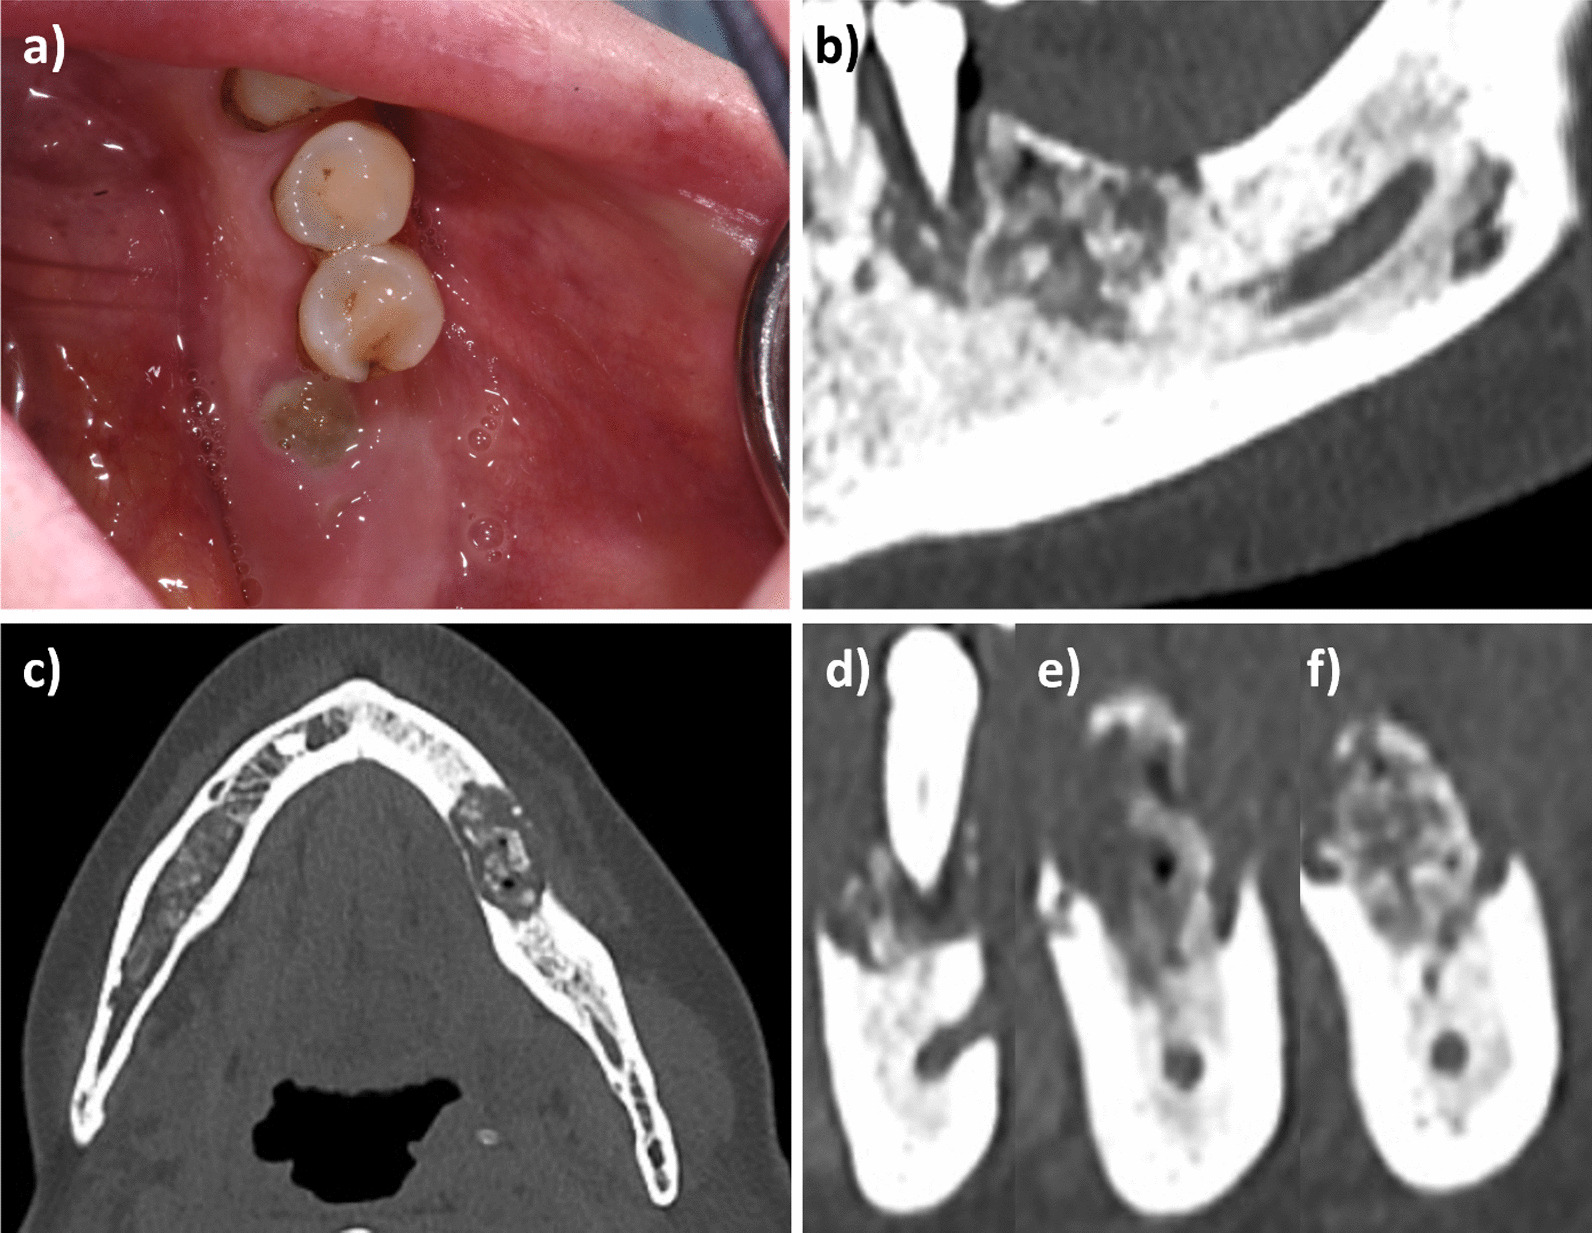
MRONJ stage 1 SICMF-SIPMO, lower jaw: a clinical view; b–f CBCT scan sections
